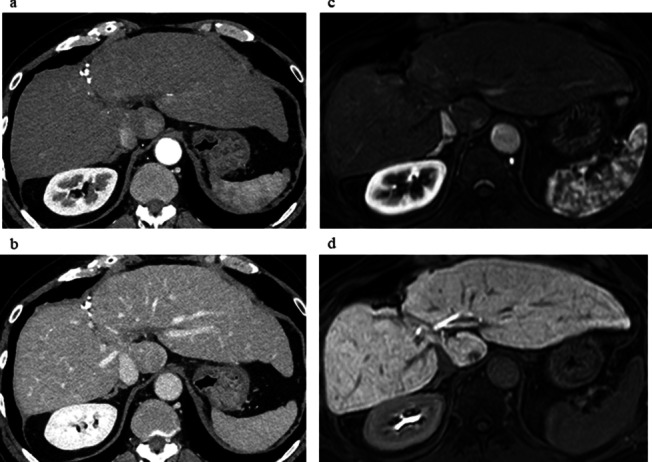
Preoperative imaging observations. Contrast-enhanced CT reveals the tumor in the caudate lobe. The tumor was slightly enhanced at the arterial phase (a), and washed out at the venous phase (b). MRI contrasted with gadoxetate sodium also shows the tumor in the caudate lobe (arterial phase (c) and hepatobiliary phase (d))
